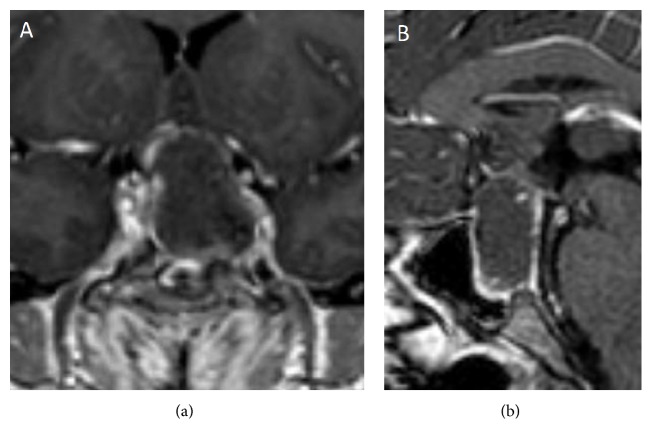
Coronal (a) and sagittal (b) T1-weighted MRI images after gadolinium administration show a moderate “ring” enhancement in a patient with pituitary apoplexy.
